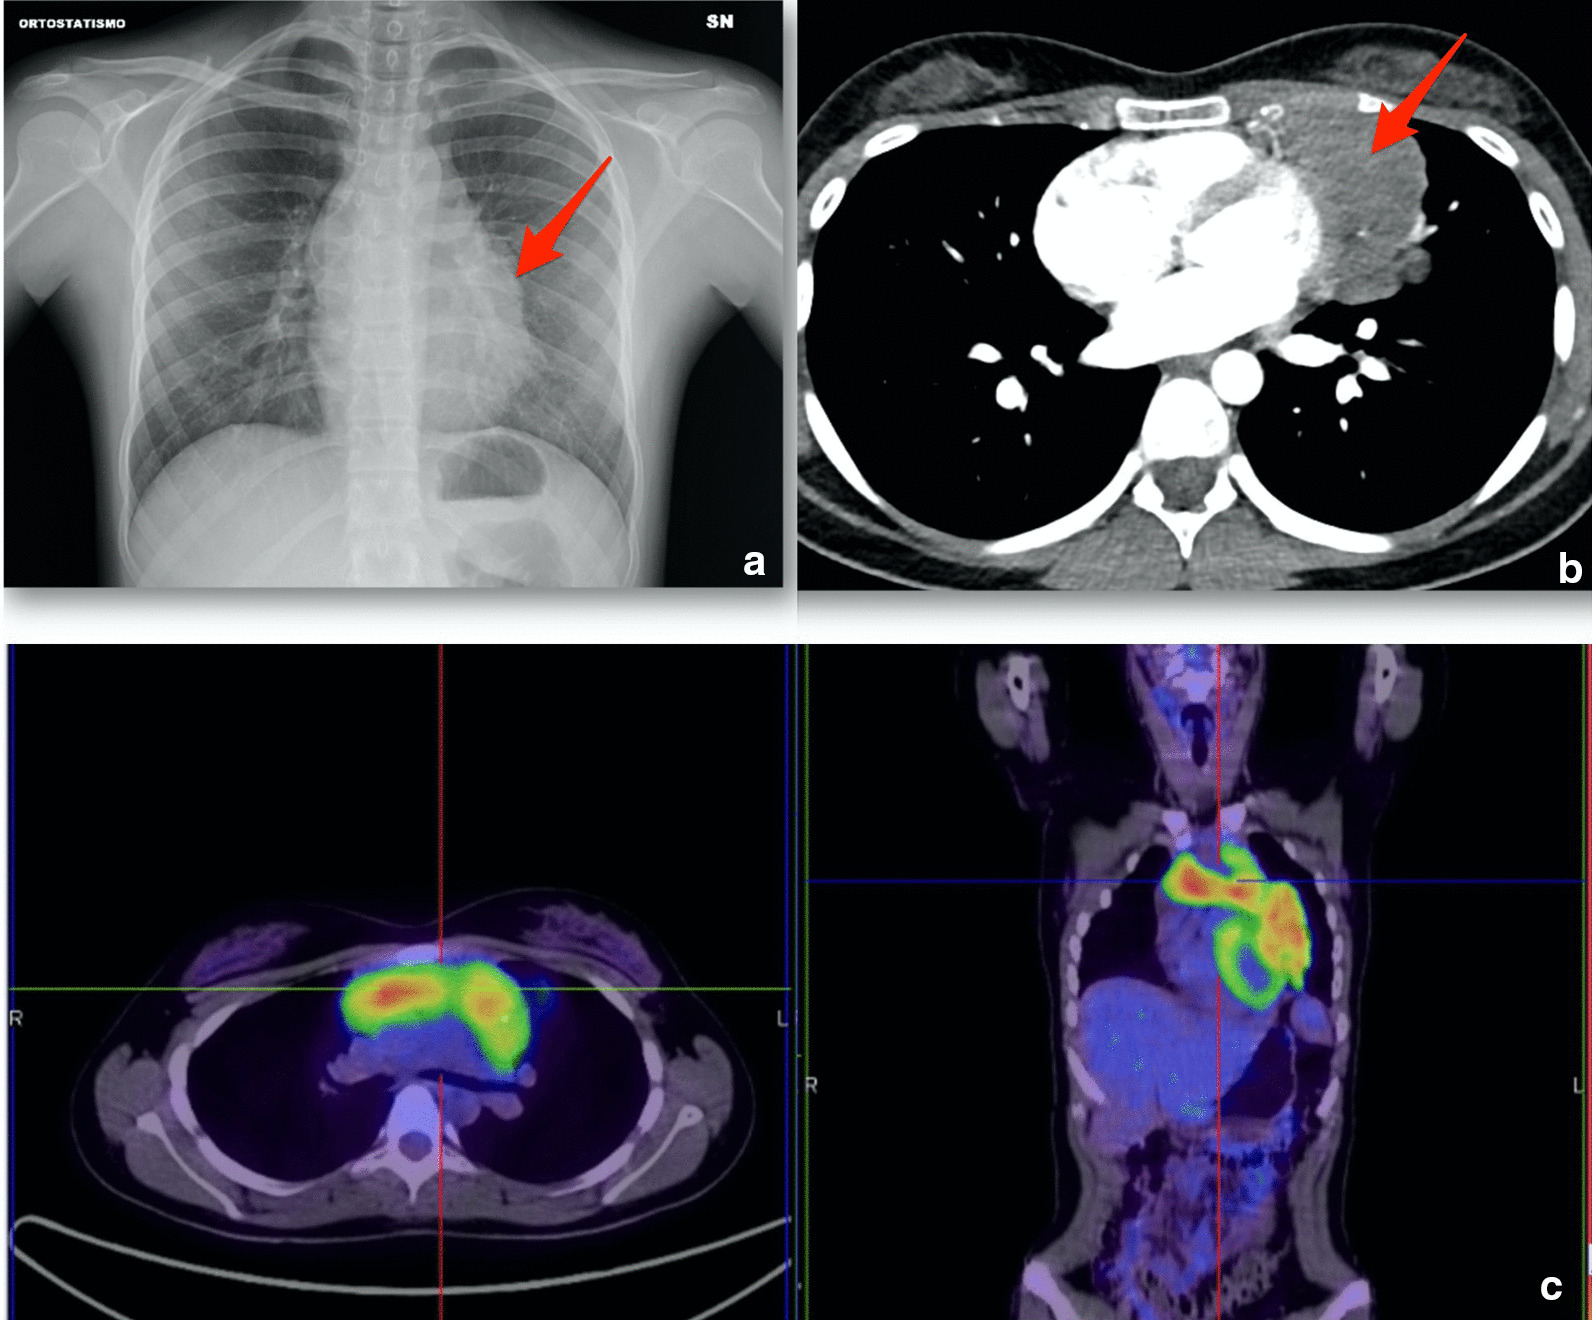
Patient imaging. a Left chest X-ray with enlarged mediastinum (see arrow). b Chest computed tomography scan showing the mediastinal mass (see arrow). c
